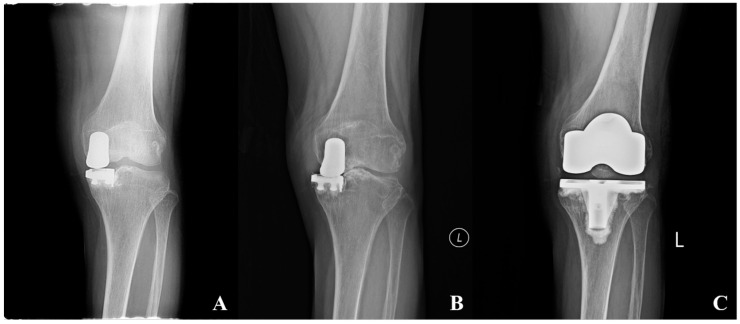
Revision to total knee arthroplasty for polyethylene wear and osteoarthritis progression after medial fixed-bearing unicompartmental knee arthroplasty (UKA). A 64-year-old female patient who underwent revision total knee arthroplasty (TKA) following medial fixed-bearing UKA in the left knee. (A) Postoperative anteroposterior radiograph after medial fixed-bearing UKA. (B) Knee anteroposterior radiograph at 13.3 years postoperatively demonstrating polyethylene wear and osteoarthritis progression. (C) Postoperative anteroposterior radiograph after revision to TKA. The revision was performed as a primary TKA without the use of stems or metal blocks.
